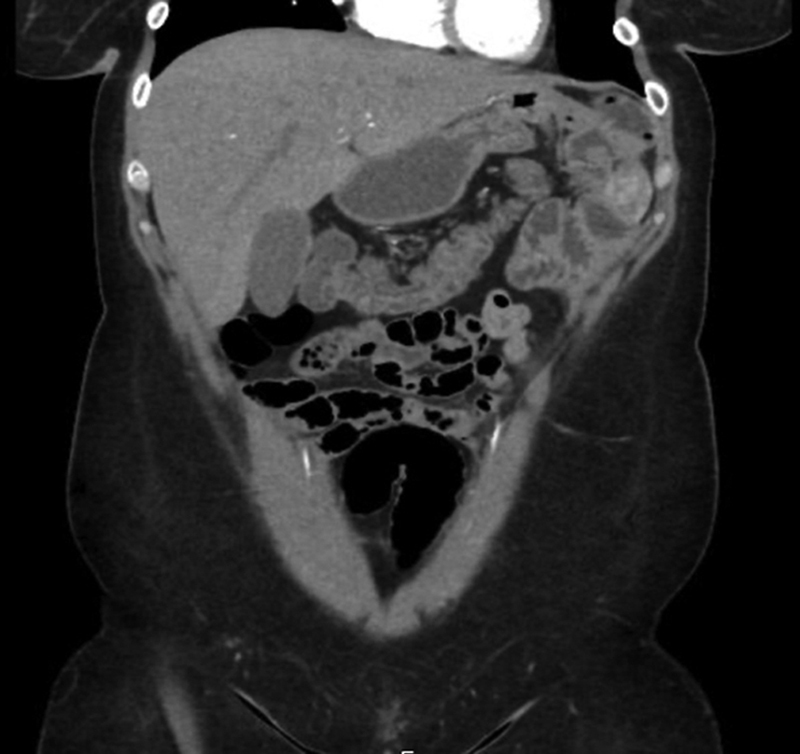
Computed tomography (CT) demonstrating distended excluded stomach (case 3).
